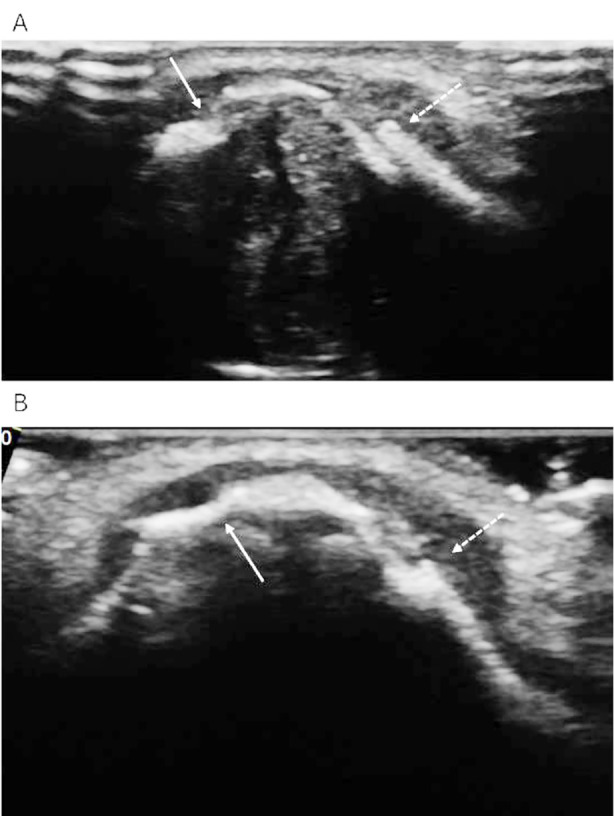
Ultrasound image of a 51-year-old male patient with compound nasal bone fracture. A: Partial angular displacement of the nasal bone, no dislocation of the broken end (solid line arrow), continuous interruption of the hyperechoic zone of the bone cortex, dislocation and separation of the broken end, overlapping each other (dotted line arrow). B: After reduction of nasal bone fracture, the fracture ends were well aligned (solid arrow), the fracture ends were moderately aligned and the periosteum was rough (dotted arrow).
